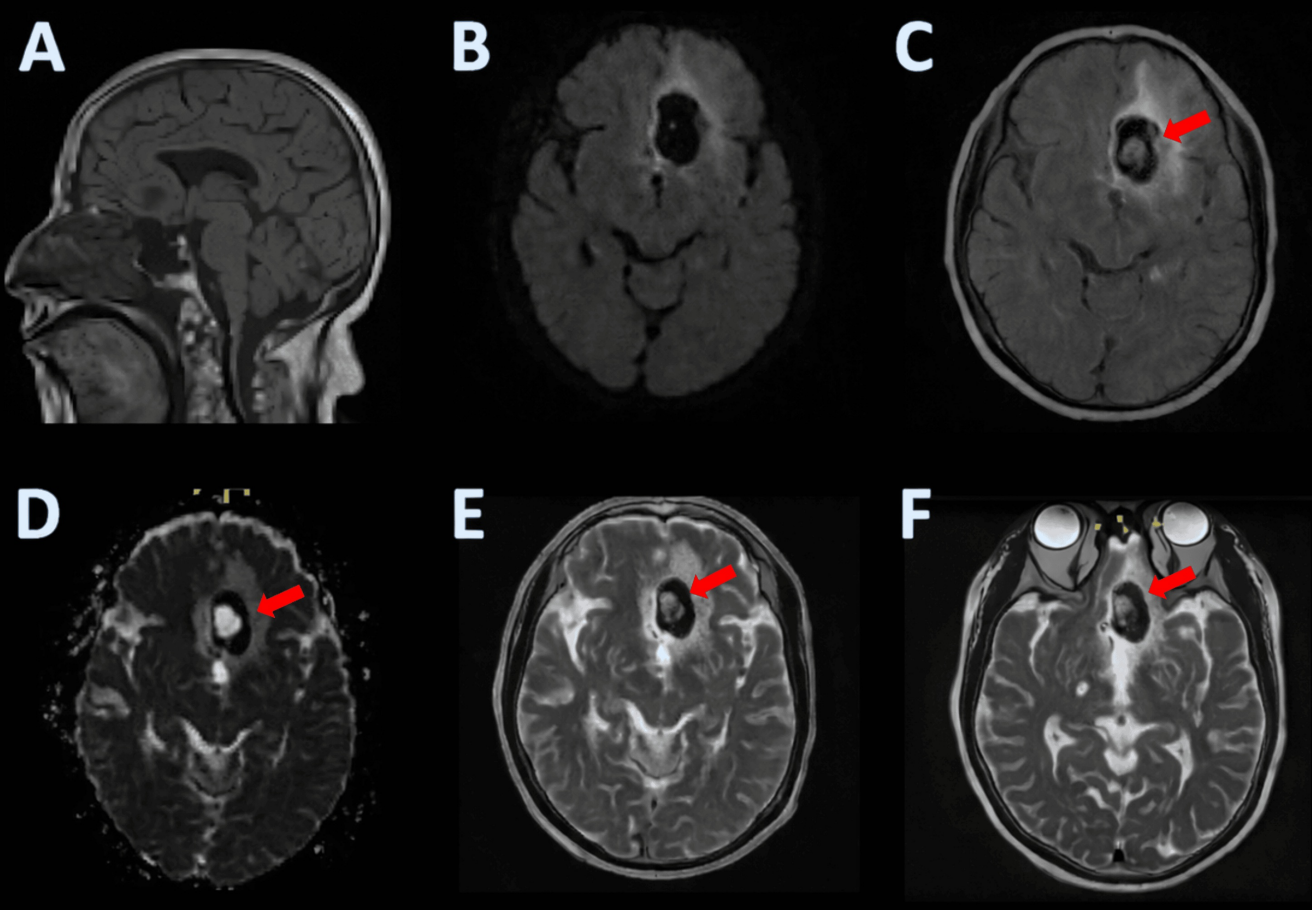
Brain MRI. (A) Sagittal T1-weighted image showing the left frontal hemorrhagic cystic lesion. (B) Axial DWI demonstrating restricted diffusion along the left cingulate region. (C) Axial FLAIR image highlighting the cystic lesion with surrounding edema; the red arrow marks the eccentric intracystic nodule suspicious for a scolex. (D) ADC map corresponding to areas of restricted diffusion, with the red arrow indicating the intracystic nodule. (E) Axial T2-weighted image demonstrating the internal hyperintense cystic component and the intracystic nodule (red arrow). (F) Axial T2-weighted image showing adjacent hemorrhagic changes and the intracystic nodule marked by a red arrow. DWI, diffusion-weighted imaging; ADC, apparent diffusion coefficient; FLAIR, fluid-attenuated inversion recovery
